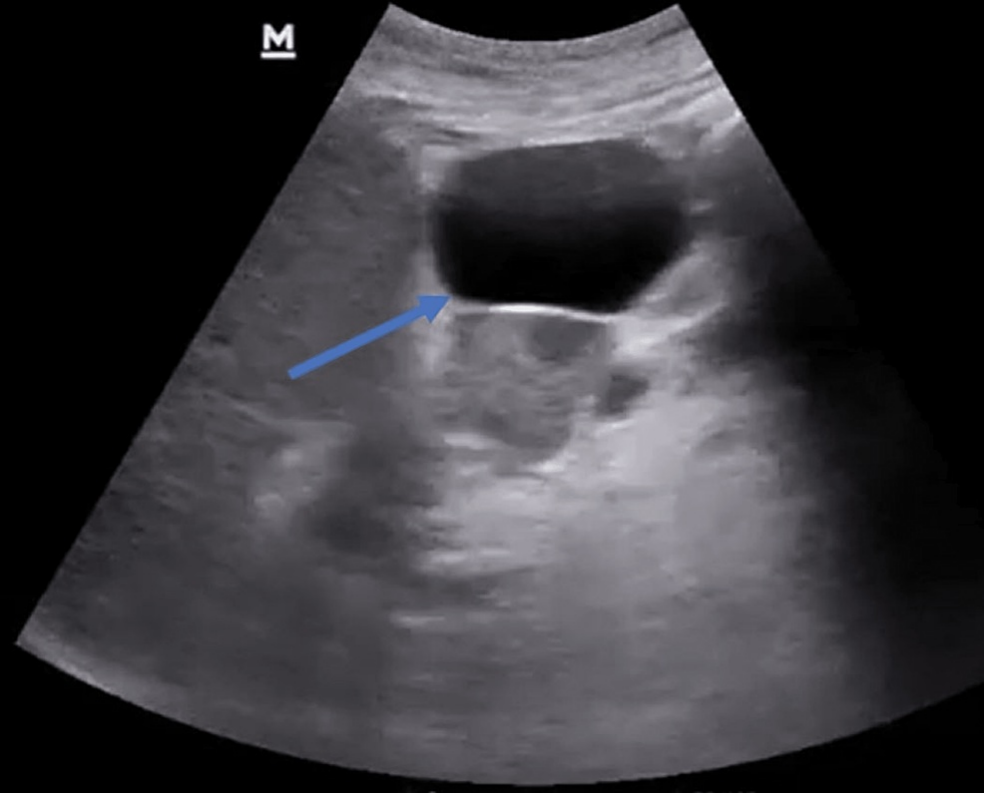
Abdominal ultrasonography demonstrating a well-defined cystic lesion in the liver.
